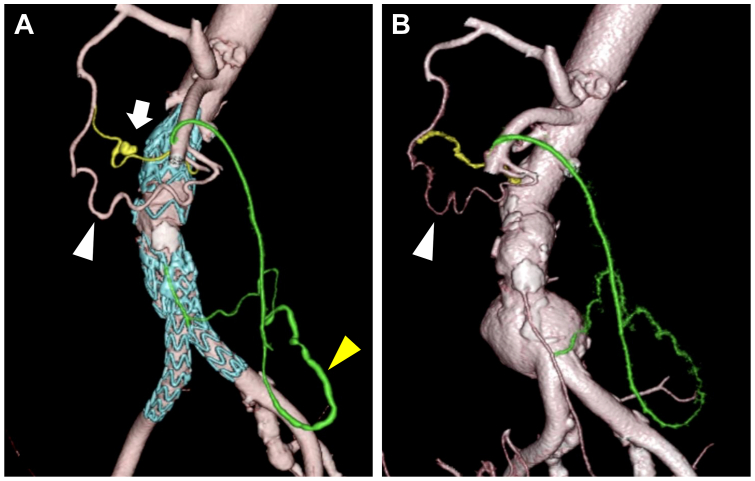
(A) Emergency three-dimensional computed tomography (3D-CT) scan showing the vasodilation from the superior mesenteric artery (SMA) to the Riolan arcade (*yellow arrowhead*) and the pancreaticoduodenal (PD) arcade (*white arrowhead*). A pseudoaneurysm of the anterior superior PD artery (PDA) (*white arrow*) was revealed. The vessels in *green* represent the Riolan arcade from the SMA to the inferior mesenteric artery (IMA). (B) Preoperative 3D-CT showing that the PD arcade from the SMA (*white arrowhead*) was not dilated, and no obvious PDA aneurysm was detected.
